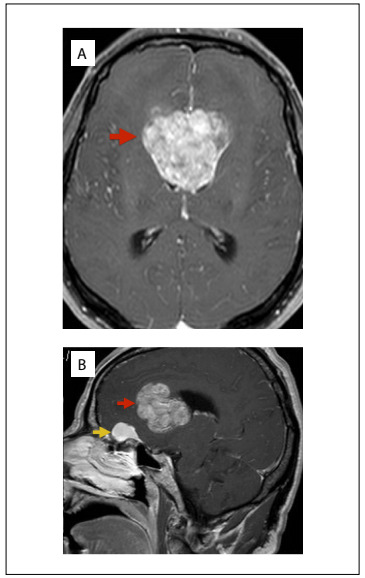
Magnetic resonance imaging of a patient with simultaneous brain tumors: A - axial image showing ventricular tumor; B - sagittal image showing two lesions: olfactory groove meningioma and ventricular tumor.
